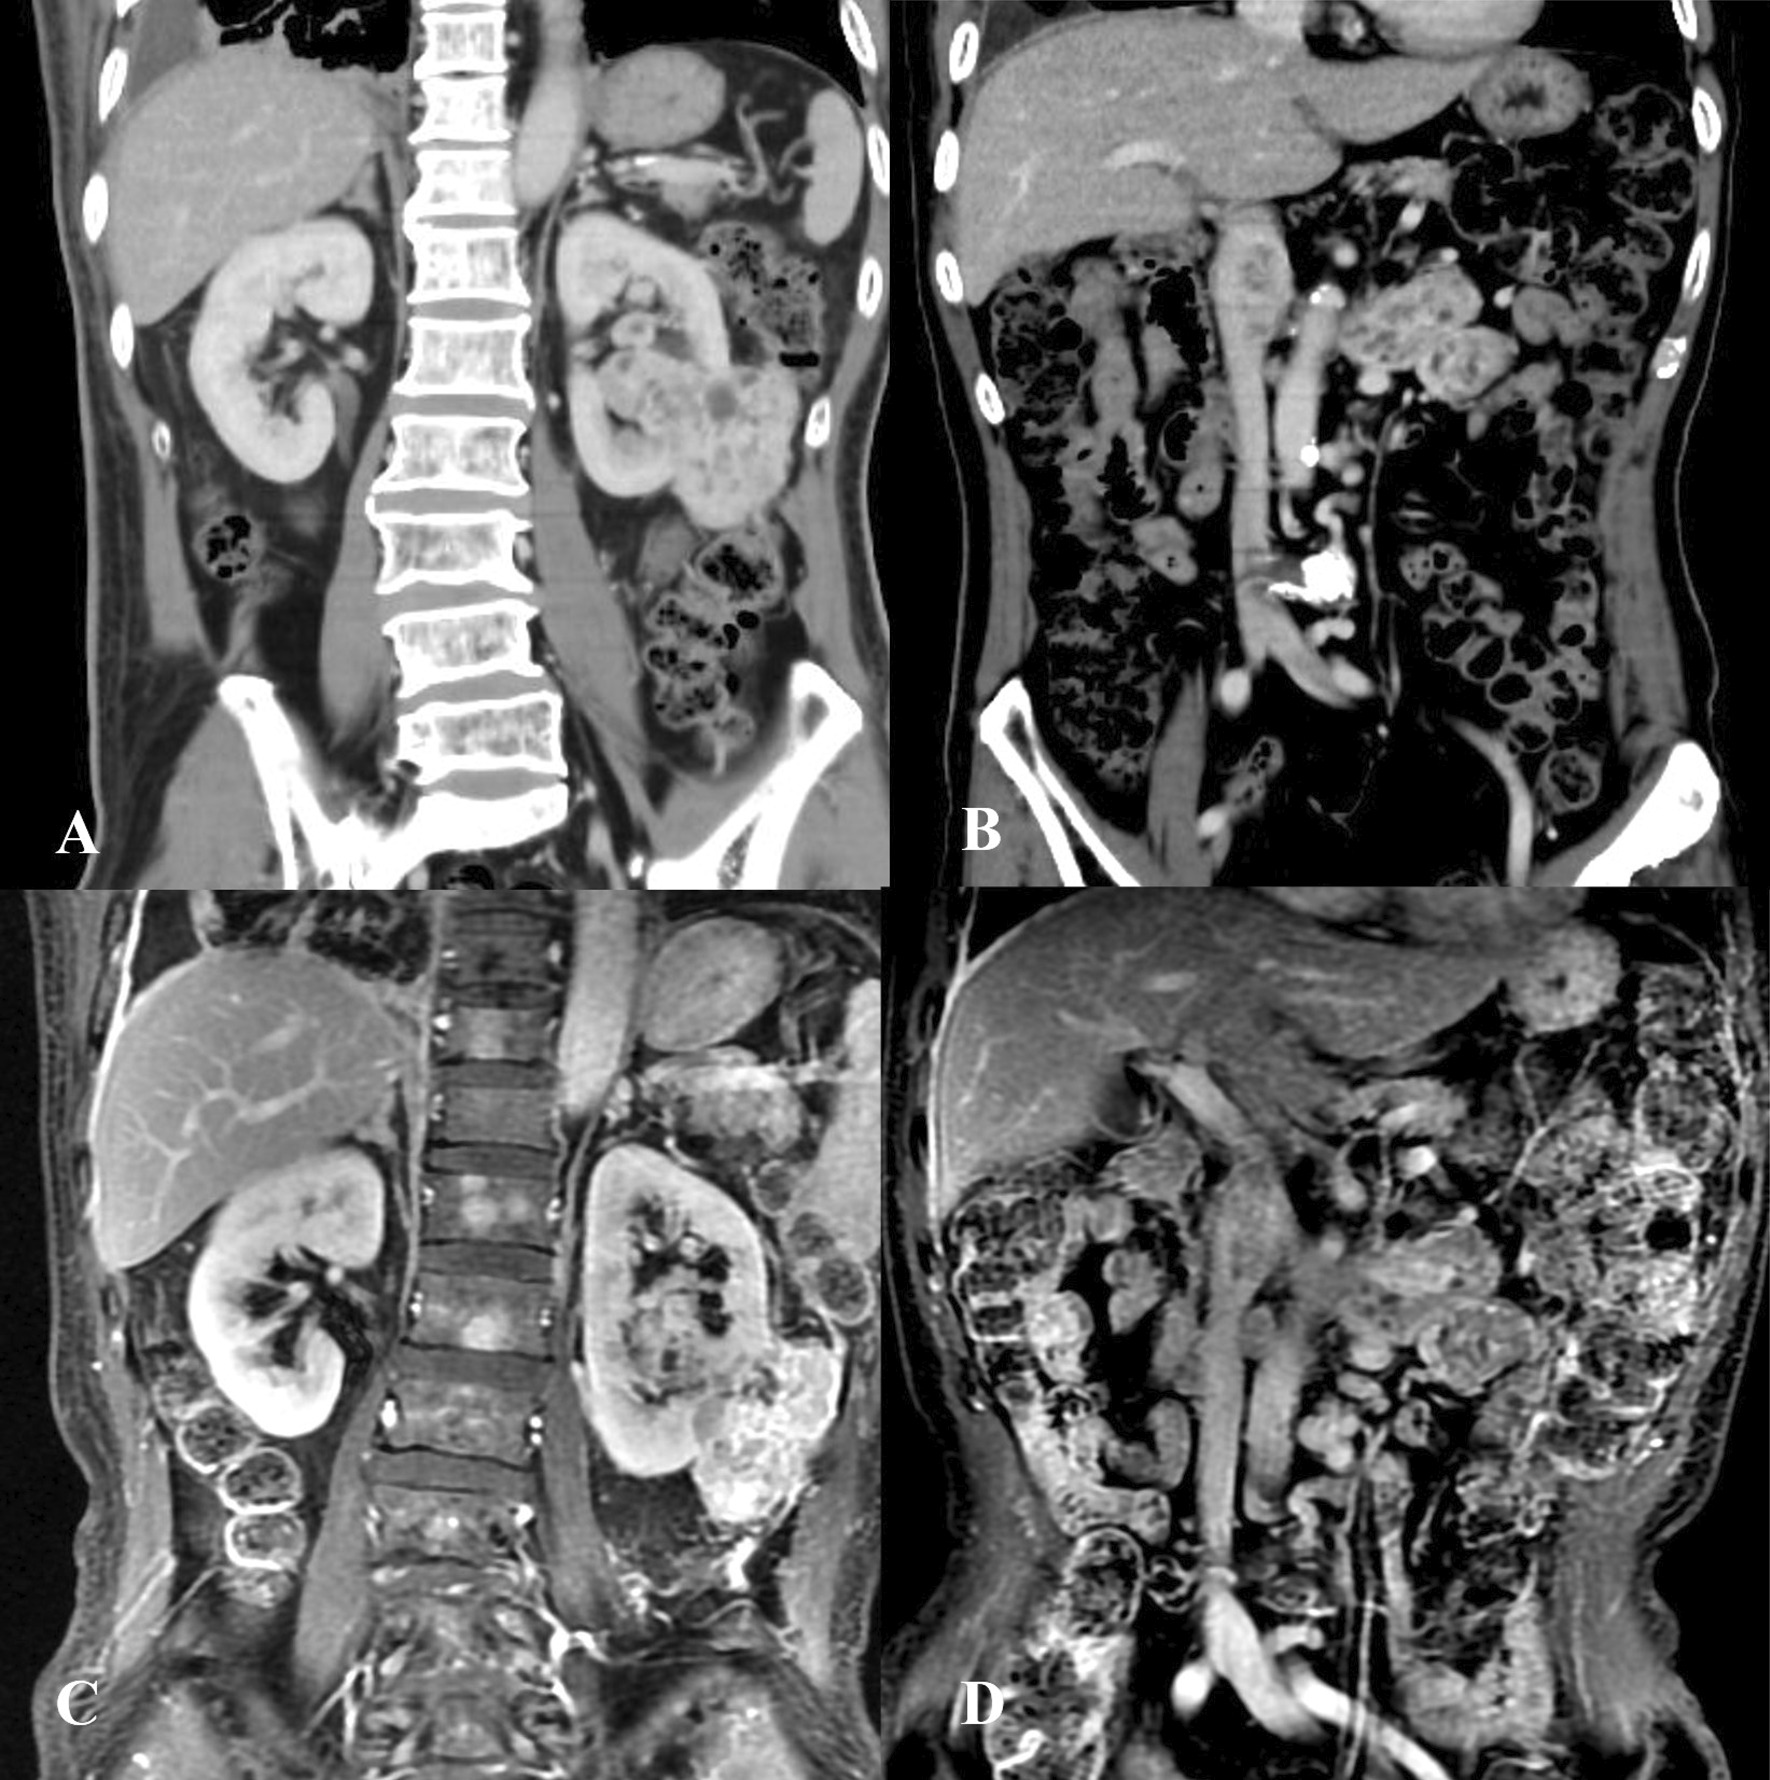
Preoperative imaging examination of a 72-year-old male patient. A Enhanced CT of the urinary system showed irregular soft tissue density of the left kidney in the range of 7.8 × 3.6 × 6.6 cm, and the enhanced scan showed uneven and obvious enhancement. Left renal cell carcinoma was considered for diagnosis. The tumor involved renal pelvis, calyces and perirenal fascia. B Soft tissue density was seen in the left renal vein and inferior vena cava, and uneven enhancement was showed on enhanced scan. The diagnosis considered tumor thrombus in the left renal vein and inferior vena cava. C Inferior vena cava enhanced magnetic resonance indicated left renal tumor. D Irregular filling defects were observed in the left renal vein and inferior vena cava, and the length of tumor thrombus in the inferior vena cava was 3.7 cm
